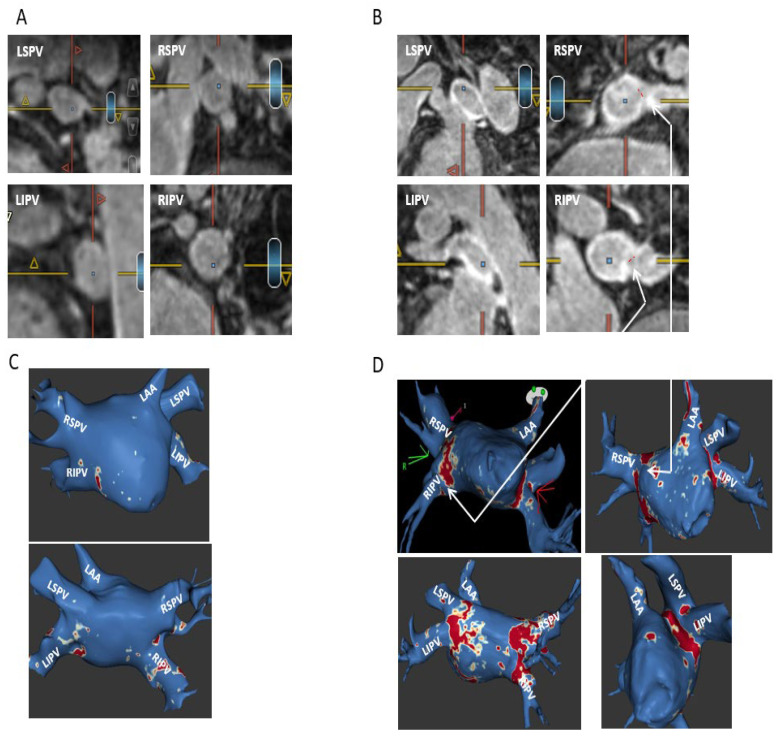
A typical patient with cryoballoon-induced complete/sub-complete circumferential fibrosis in all PVs. Shown are pre-ablation (A) and post-ablation (B) cross-sectional short-axis views of the four PVs revealing minimal PV ostial fibrosis at baseline, while at post AF ablation, there is a complete circumferential fibrosis around both left PVs and sub-complete circumferential fibrosis around both right PVs, with minimal gaps in the anterior segments of both veins (red dottedlines). (C,D) Cor-responding ADAS 3D LGE model images pre (C) and post (D) cryoballoon AF ablation showing minimal LA fibrosis at baseline, post-ablation complete circumferential fibrosis around both left PVs without any gaps, and sub-complete circumferential fibrosis around both right PVs due to minimal gaps at their anterior segments, corresponding with the original images analyses. The post-ablation fibrotic areas on ADAS are colored red, while PV gaps are seen as blue color. The arrows show the matching of the minor PV gaps around both right PVs between the original MRI cross-sectional views and the ADAS model images.
